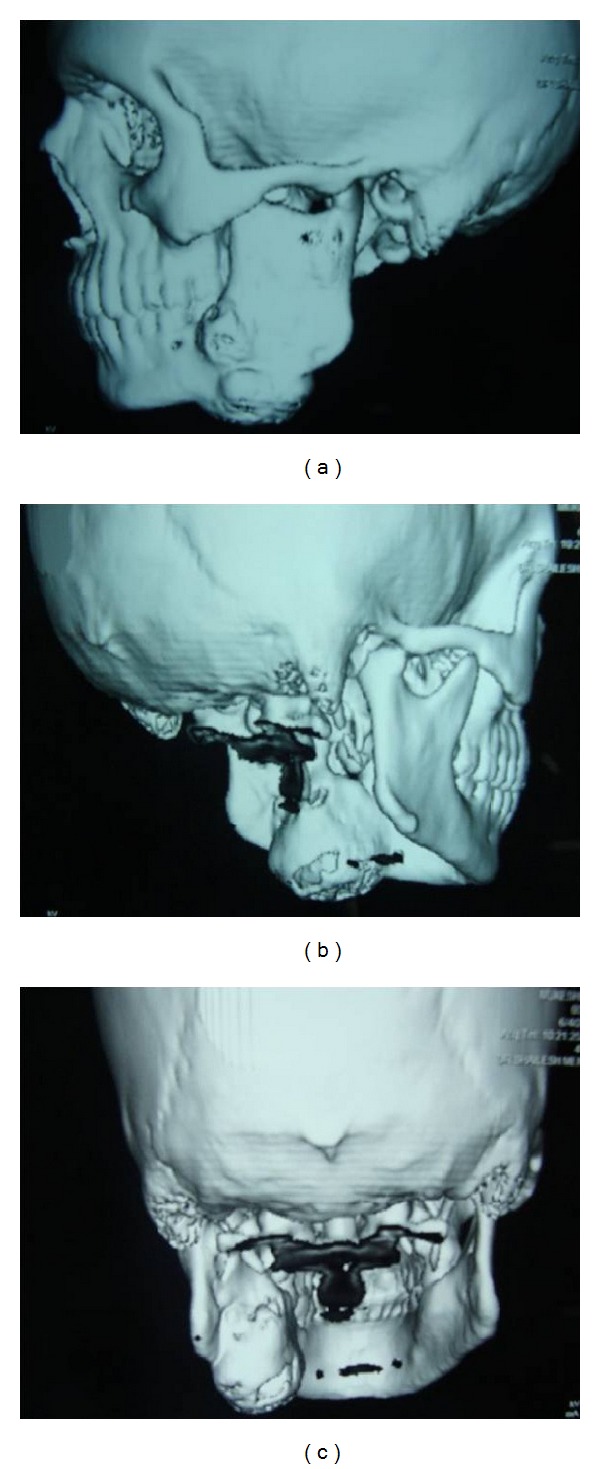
3D CT image showing the lesion in the body and ramus of the mandible, (a) left lateral surface, (b) lingual surface of left mandible, and (c) lingual surface of mandible from the posterior view.
